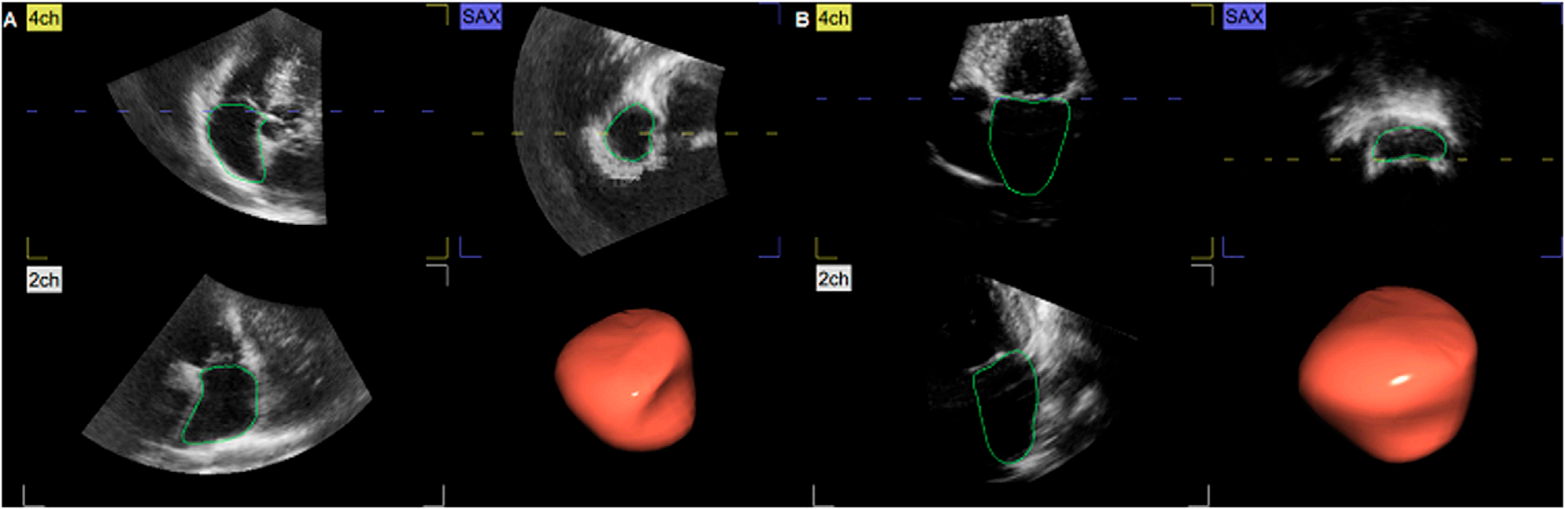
Three-dimensional (3D) echocardiographic assessment of the right atrium (RA) (A) and left atrium (LA) (B). From the apical view, both transversal and longitudinal visualization of atrial volume changes during the cardiac cycle was obtained, with endocardial contouring and cast reconstruction. 2ch, two-chamber view; 4ch, four-chamber view; SAX, short-axis view.
